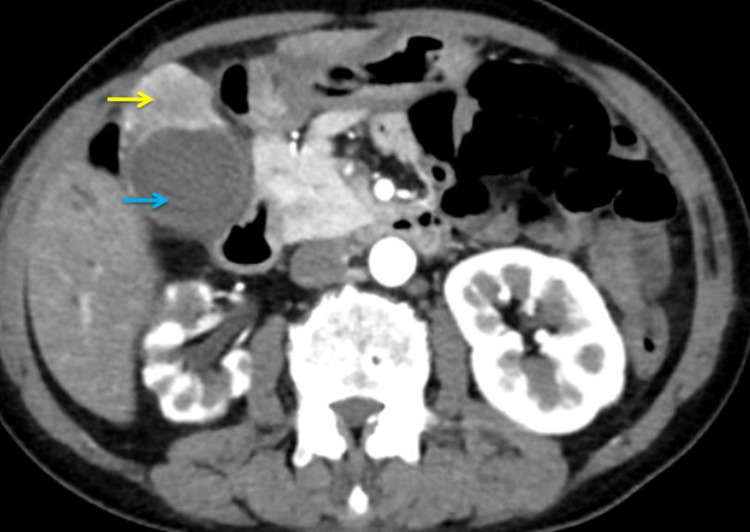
Computed tomography of the abdomen (axial view) showing well-defined enhancing tumor in the gallbladder (yellow arrow), involving segment V of the liver and abdominal wall, with distended gallbladder (blue arrow) without any evidence of cholelithiasis.
